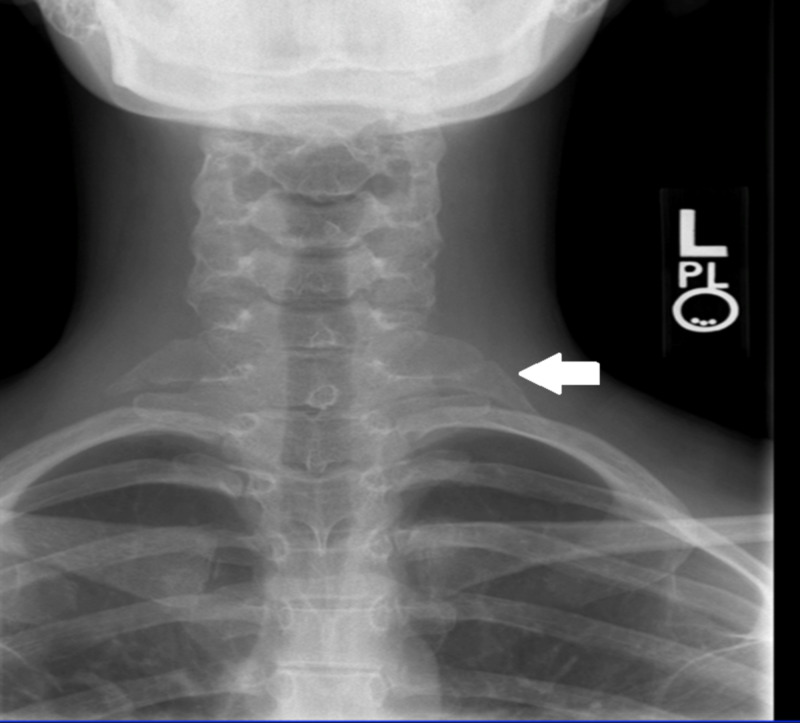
Cervical rib Radiograph of the cervical spine showing a left cervical rib originating at the articulation of C7 and T1 (white arrow).
